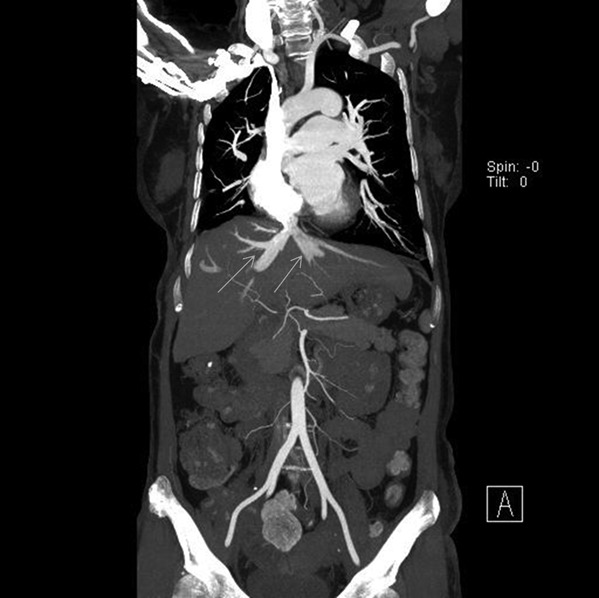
Computed tomography (CT) coronal view demonstrated the hepatic veins draining directly into the right atrium (red arrow).
